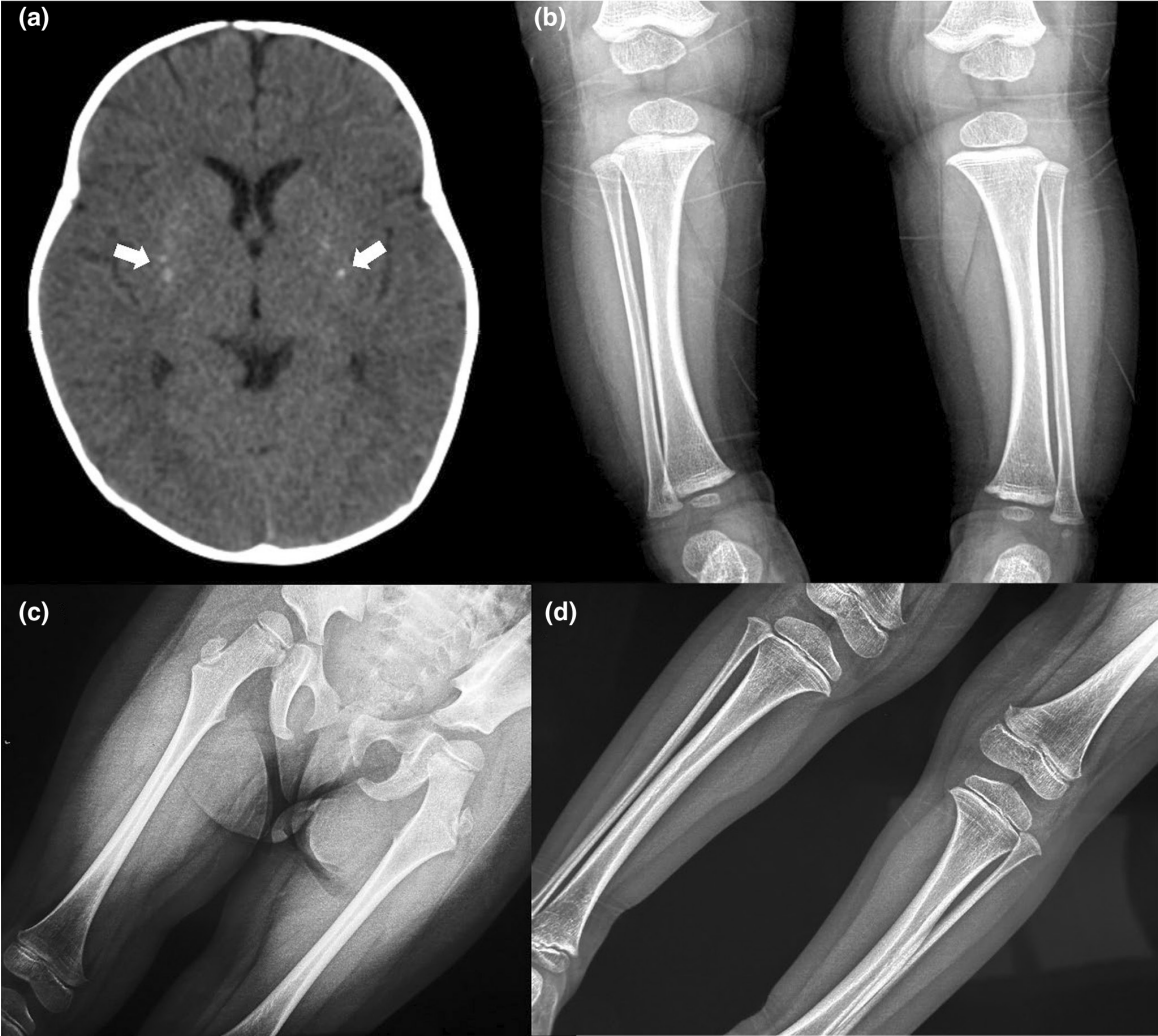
Radiographic studies of probands. (a) Brain computed tomography of F1‐II‐1. The arrowheads indicate calcifications in the basal ganglia. (b–d) X‐ray images show tubular long bones with cortical thickening and medullary stenosis in F1‐II‐1 (b) and F3‐II‐1 (c, d).
